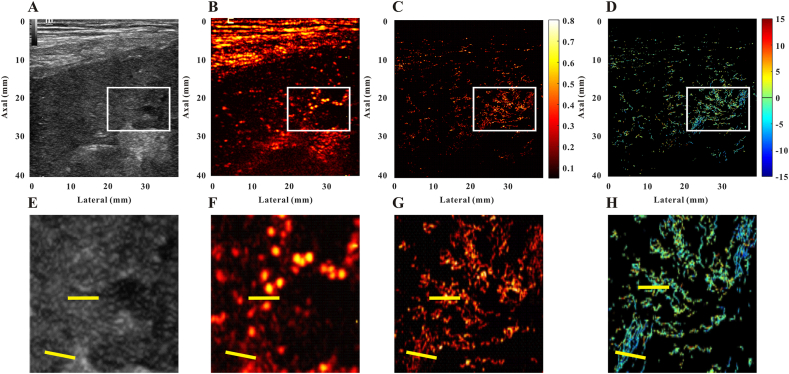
Representative images of an allograft dysfunction patient. B-mode image (A), contrast-enhanced ultrasound image (B), super-resolution image (C), super-resolved velocity image (D), and the zoomed-in regions indicated as the white box of the B-mode image (E), contrast-enhanced ultrasound image (F), super-resolution image (G), super-resolved velocity image (H). Yellow lines highlight the resolution improvement among the images.
